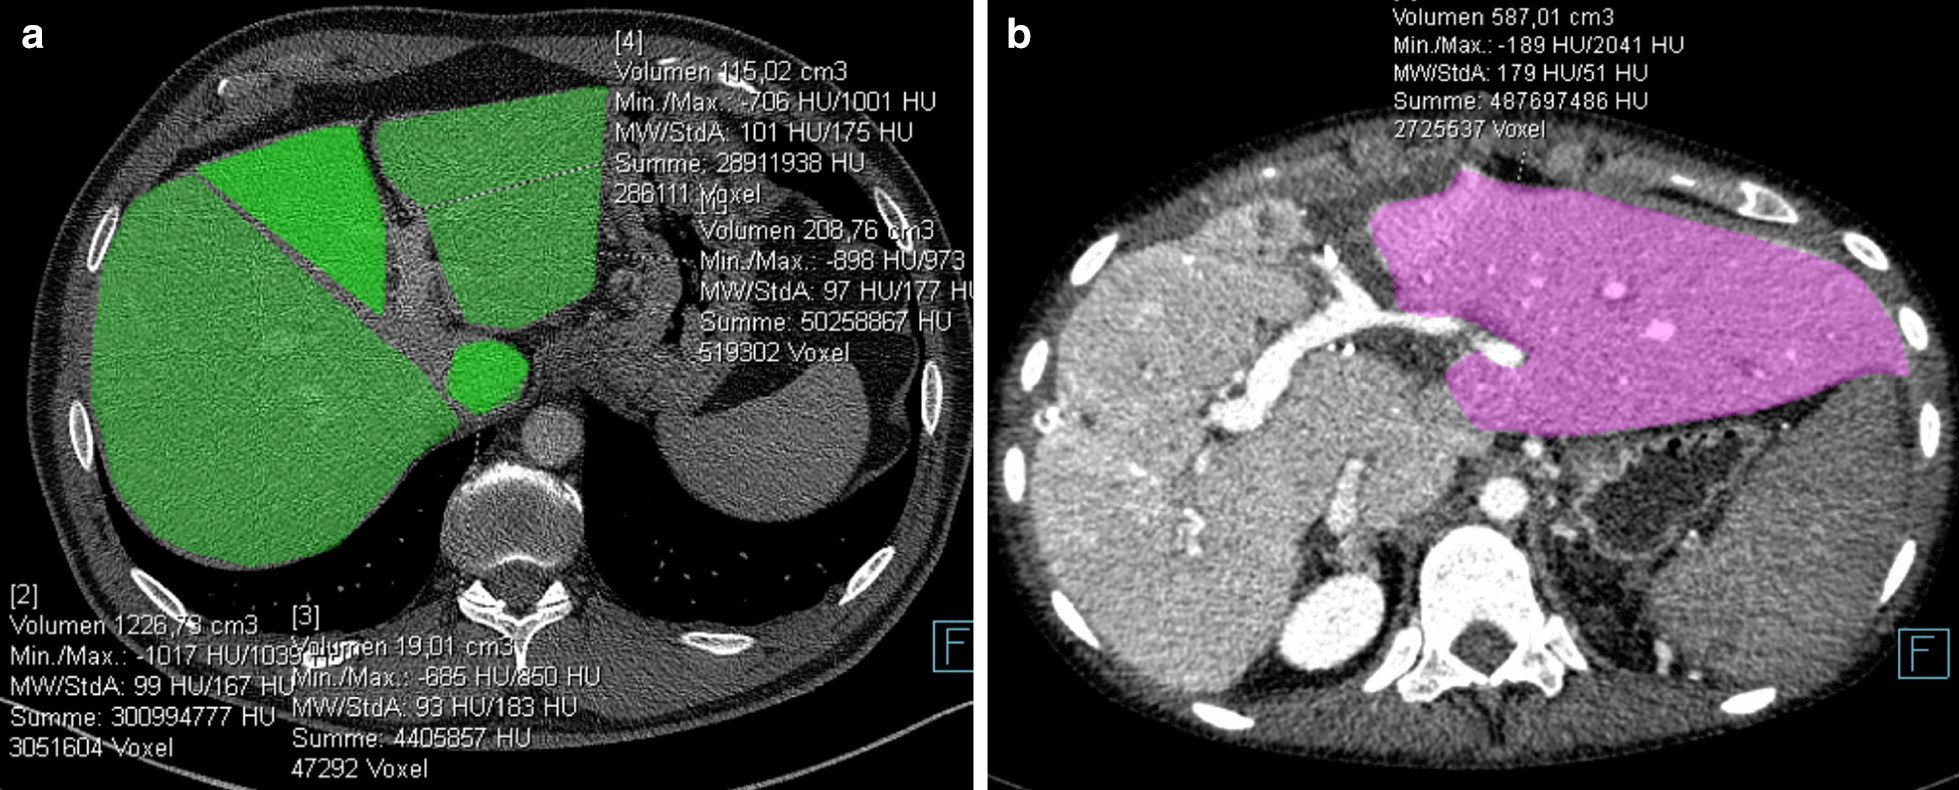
Preoperative CT scan of donor 1. a Volumetry of liver segment II/III (209 cm^3^), segment IV (115 cm^3^), segment I (19 cm^3^) and segments V–VIII (1227 cm^3^). b CT scan of recipient 1 at postoperative day 12 (before removal of the native right liver) with volumetry of the graft (587 cm^3^) after hypertrophy
